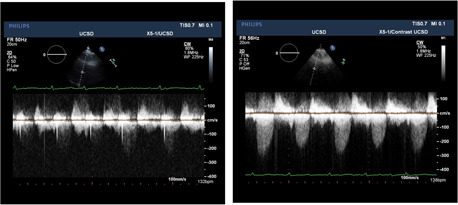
Left Panel: the aortic stenosis jet recorded from a patient with poor ultrasound transmission. Right Panel: the aortic stenosis jet after contrast injection; a jet velocity of approximately 250 cm/s is seen.
